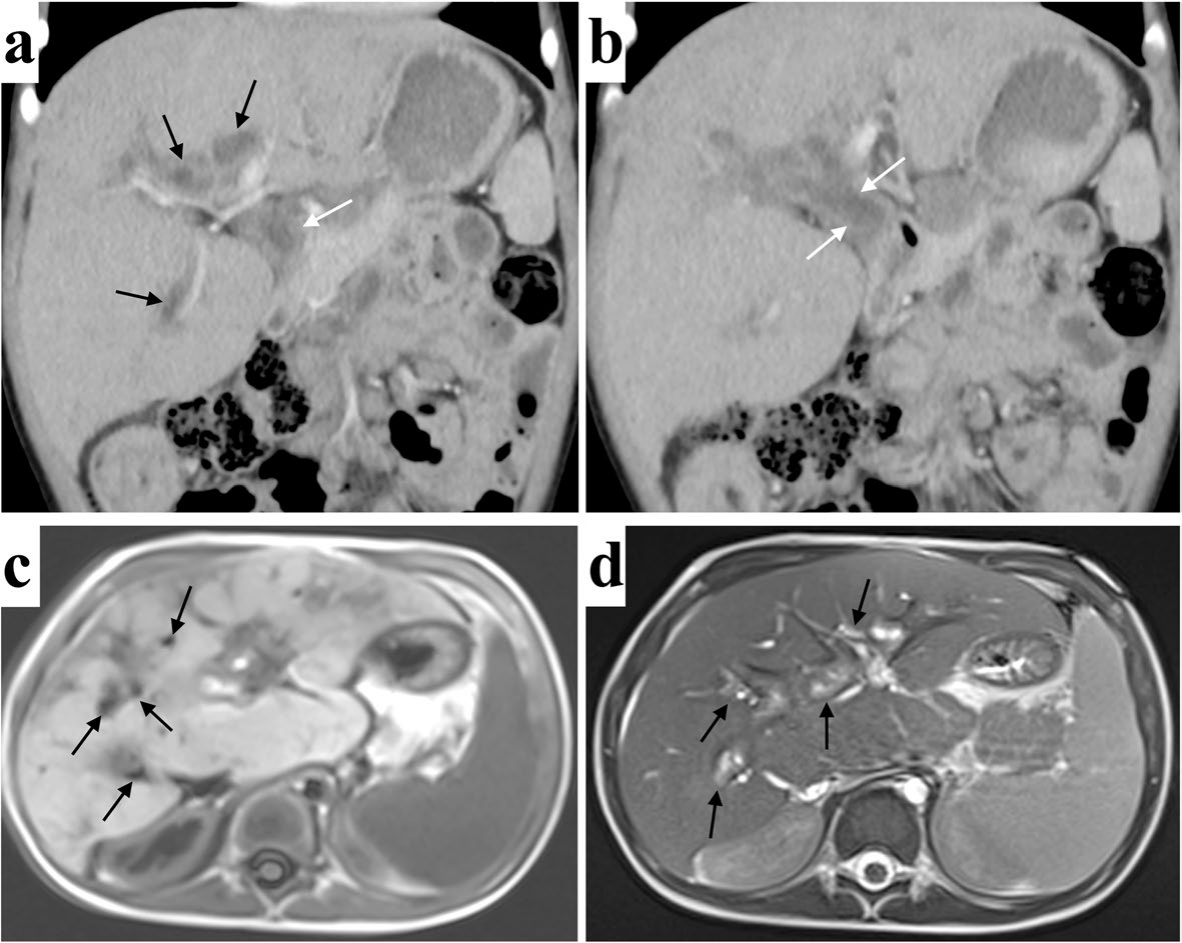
Contrast-enhanced computed tomography (CT) (coronal view) and Magnetic resonance imaging (MRI) of the patient’s abdomen. a The dilated intrahepatic bile duct (black arrows) and extrahepatic bile duct (white arrows) in CT. b The dilated extraheaptic bile duct (white arrows) in CT. c The dilated intrahepatic bile duct (black arrows) in MRI T1-weighted image. d The dilated intraheaptic bile duct (black arrows) in MRI T2-weighted image
